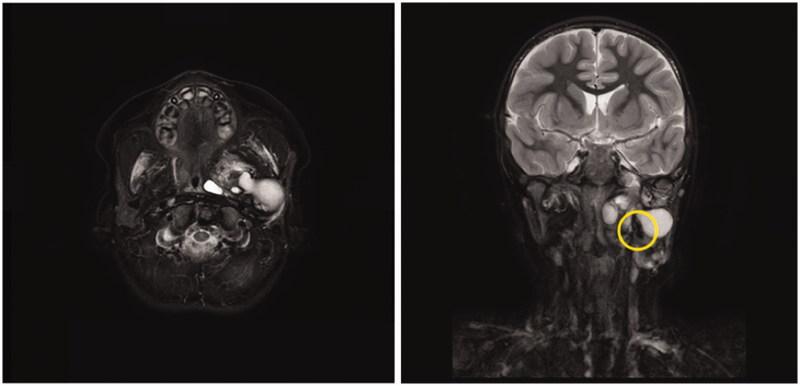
Short-tau inversion recovery (STIR) MRI at 5 years old. Majority of cystic components had shrinked. Hypo-intense structure beside the cyst (circle) was figured out to be calcification during operation later.
